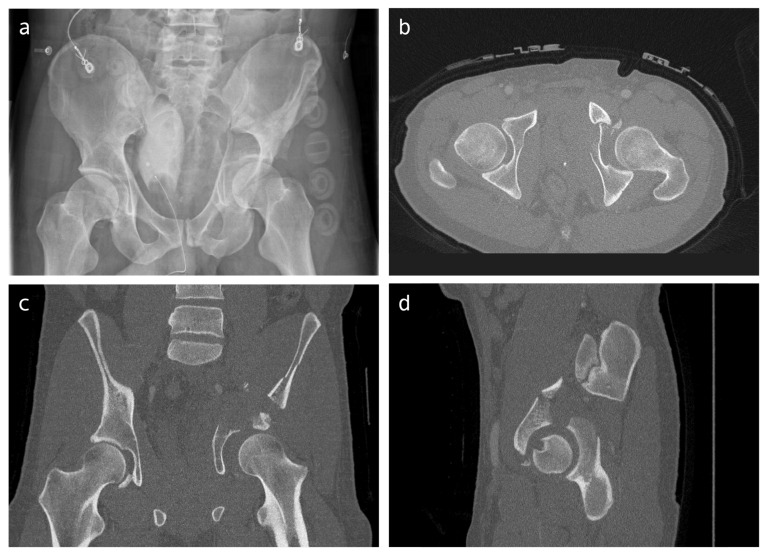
Conventional imaging provided within the surveys: (a) pelvic radiograph (AP overview), (b–d) examples of the axial, coronal, and sagittal CT slice (surgeons could scroll through every slice of the CT scan).
